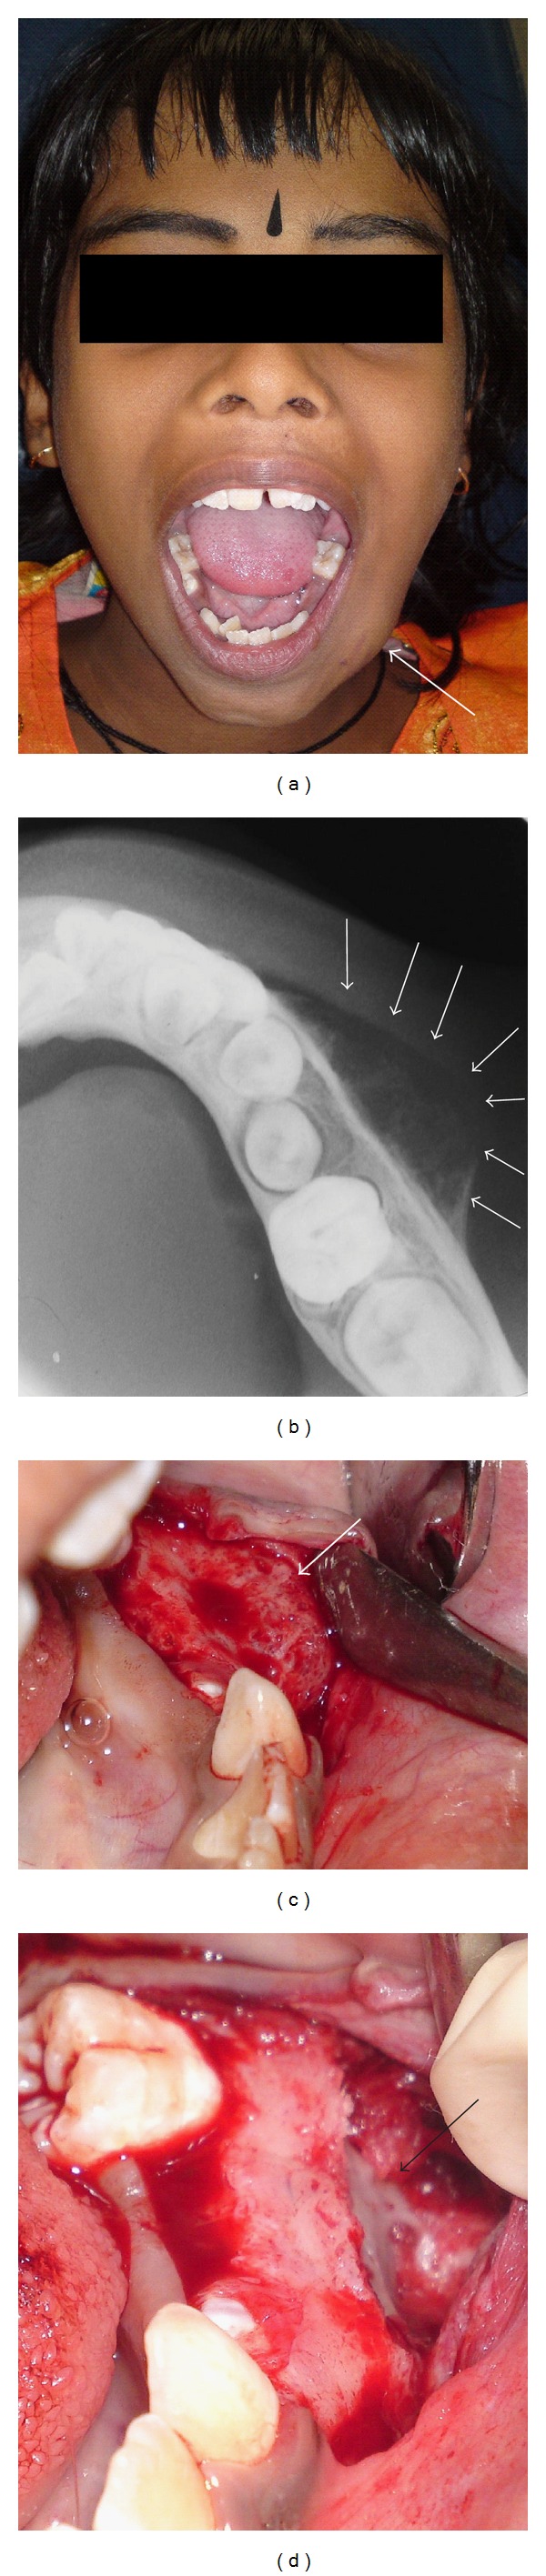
(a) Swelling of the mandible of 2-month duration. (b) Mandibular occlusal radiograph showing intact cortical plate and periosteal bone formation (arrows). (c) On surgical exposure, bony lesion seen. (d) Bone removed for biopsy.
